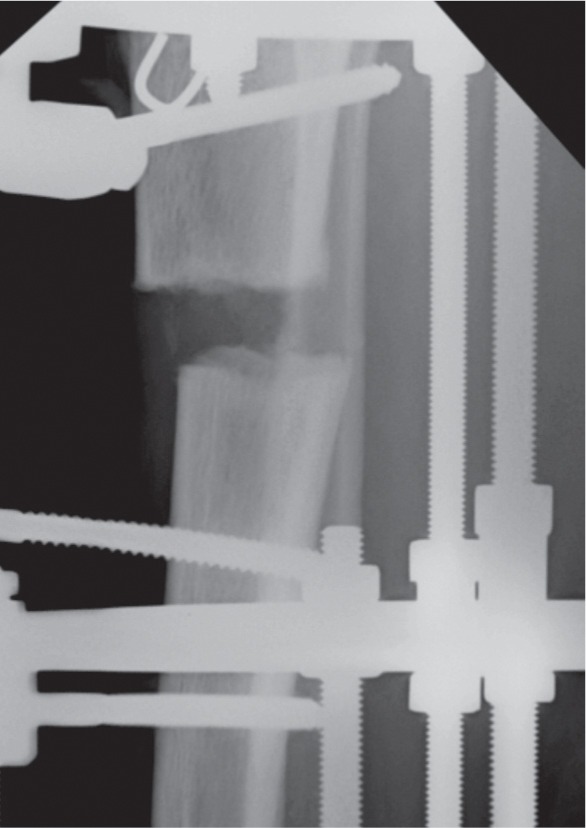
Radiograph showing Type I corticotomy with clean transverse cortical fracture.
